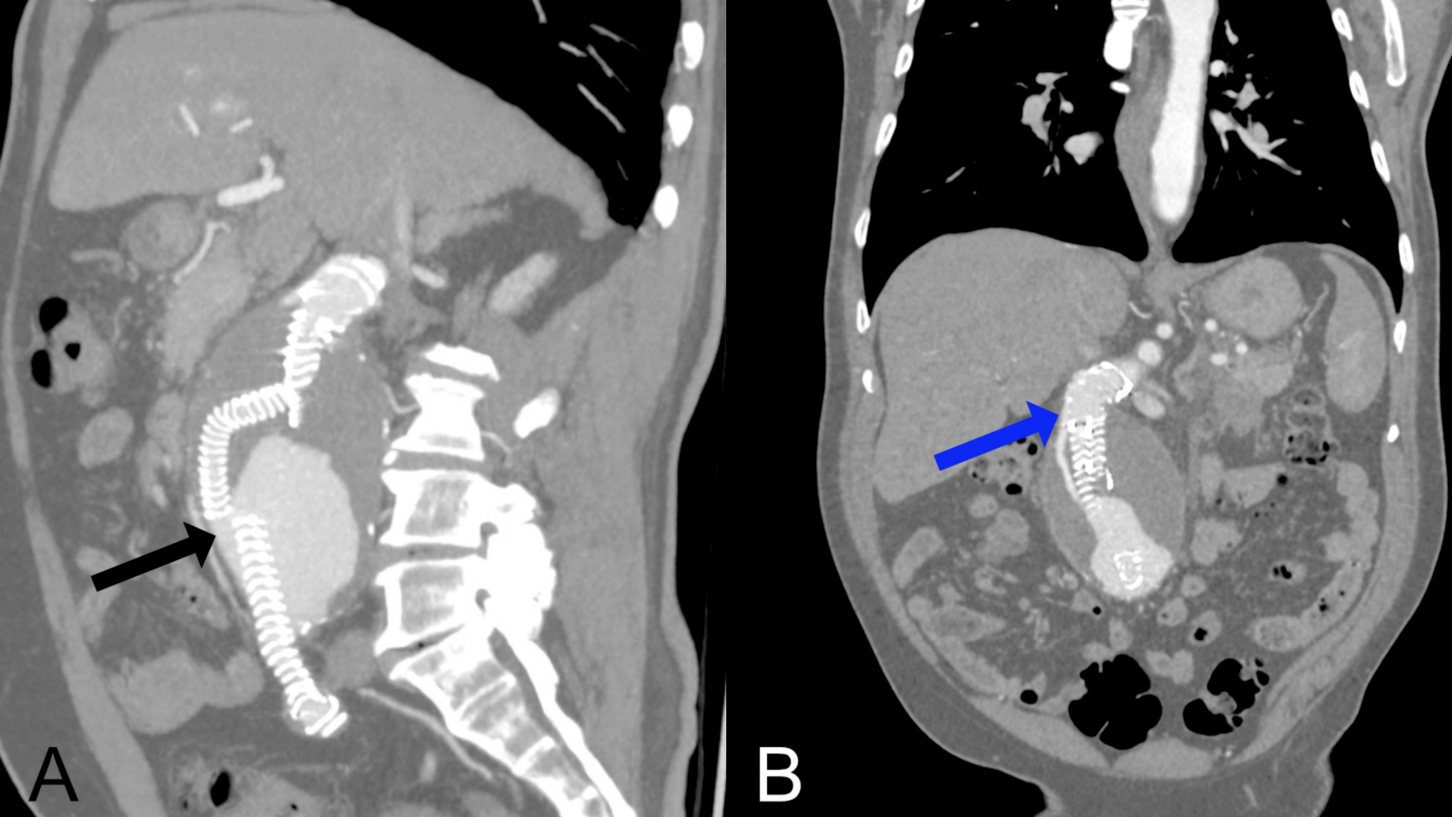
Postoperative CT angiography at presentation showing type 1a and type 3a endoleaks (A) Multiplanar reconstruction in a predominantly sagittal plane with maximum intensity projection (MIP) showing a type 3A endoleak (black arrow) caused by disconnection between the limb extension and the branch of the Anaconda bifurcated endograft. (B) Multiplanar reconstruction in a mainly coronal plane with MIP demonstrating a type 1A endoleak (blue arrow) originating from the proximal seal zone and extending into the aneurysmal sac.
